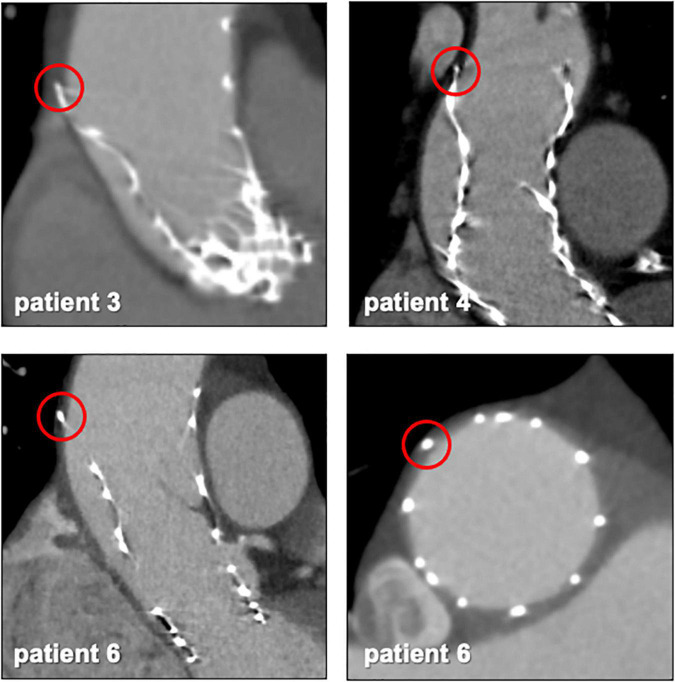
Protruding stent frames into the aortic wall. CT follow-up images from patients 3, 4, and 6 (Table 5) revealed parts of the upper crown of the stent frame protruding into the aortic wall.
